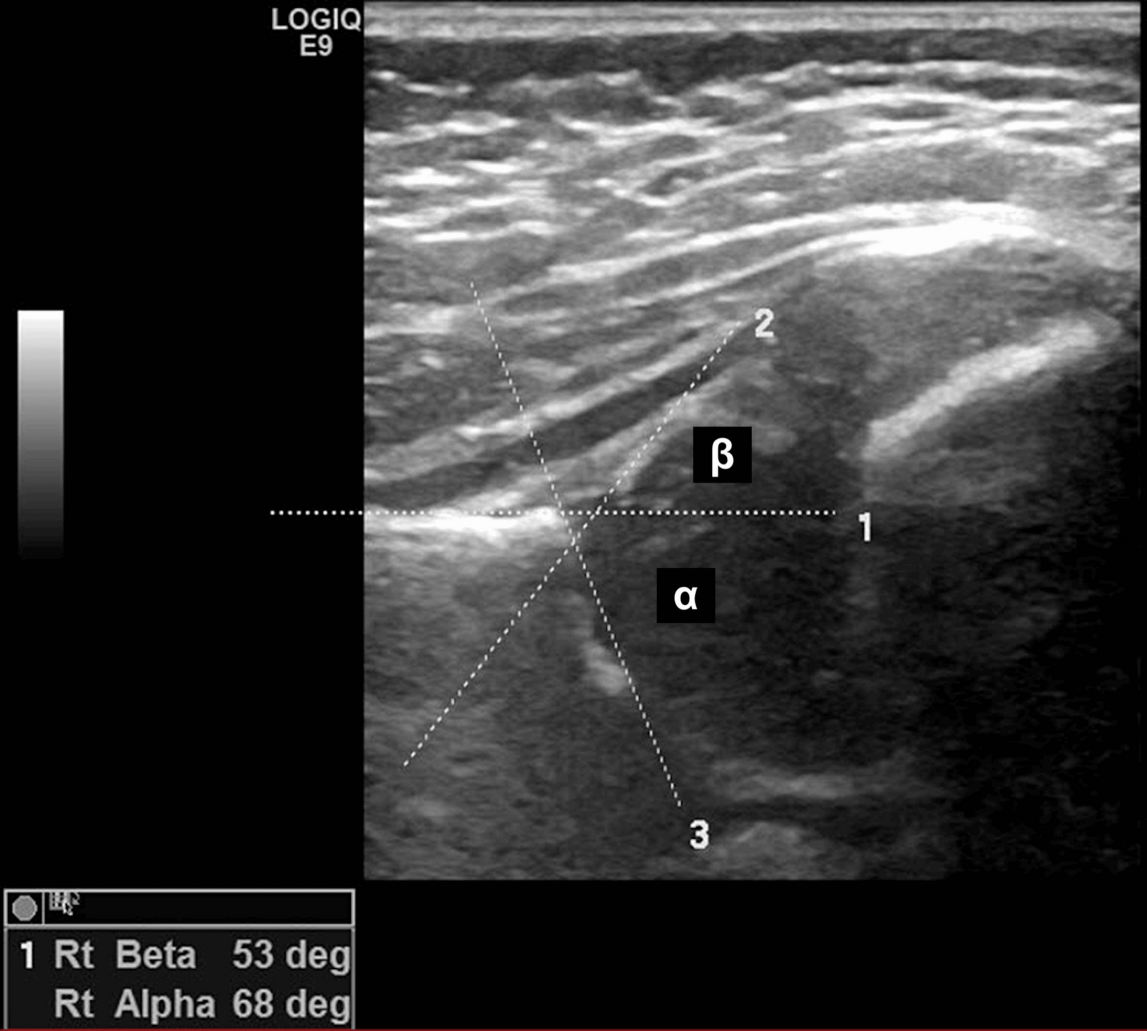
Hip ultrasound image. The image shows the correct measurement of alpha and beta angles. Ensuring the accuracy of an image involves two crucial steps. The first step is identifying eight anatomical landmarks on the image: the chondro-osseous border, femoral head, synovial fold, joint capsule, labrum, the cartilage portion of the roof, the bony portion of the roof, the bony rim (also known as the turning point between the bony roof's concavity and convexity), and the ilium. The second step is completing the usability checklist, which ensures the identification of three key elements: the lower limb of the acetabular roof (typically the brightest and most prominent lower end of the bony roof), the midportion of the ilium, and the labrum. If any of these elements are missing or not clearly visible, the sonogram is considered invalid and should not be used.
